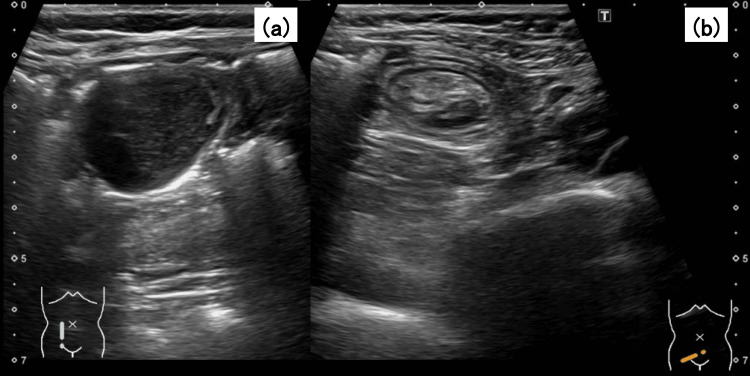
Abdominal ultrasonography: (a) A well-circumscribed, hypoechoic mass measuring 28×23×22 mm was identified in the right lower quadrant. (b) A "target sign" characteristic of ileocecal intussusception was observed
